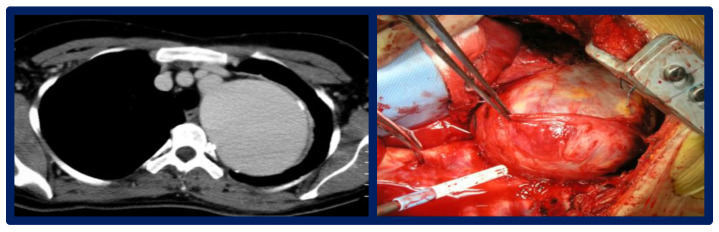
CTA axial view of a giant pCoAA; intraoperative finding.
